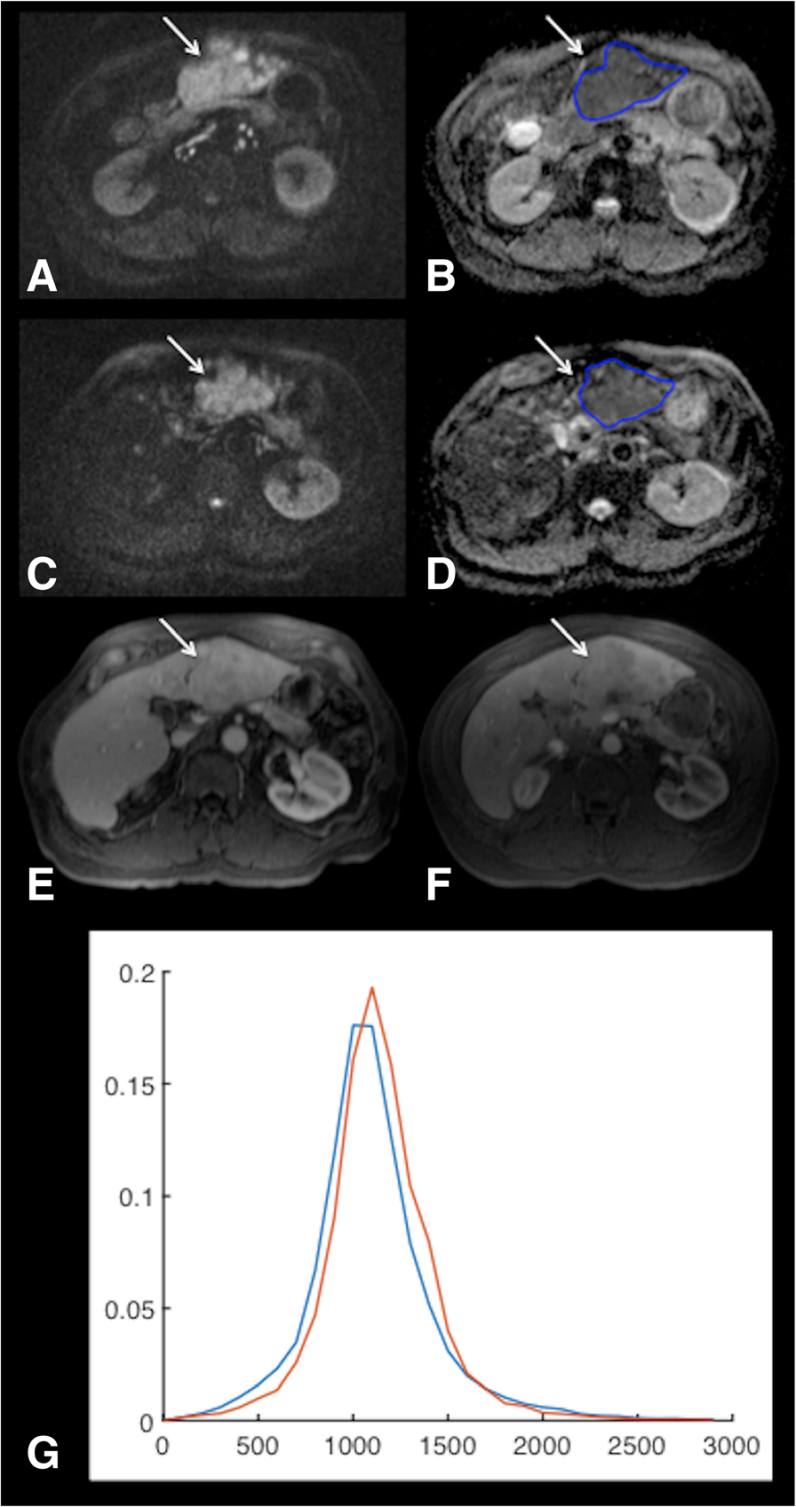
69-year-old male patient with HCV cirrhosis and HCC treated with radioembolization (RE). Pre-treatment MRI: HCC (arrows) demonstrates restricted diffusion on high b value DWI (b 800) (a) and ADC map (b). Post-treatment MRI at 6w post RE shows unchanged diffusion restriction on DWI (b 800) (c) with low ADC (d). Contrast-enhanced-T1 weighted images obtained during the portal-venous phase at baseline (e) and at 6 months (f) show stable disease at 6 months. Corresponding histogram distribution of vADC before (blue, vADC median 1.12 × 10^− 3^ mm^2^/s, vADC mean 1.12 × 10^− 3^ mm^2^/s) and 6w after RE (red, vADC median 1.17 × 10^− 3^ mm^2^/s, vADC mean 1.19 × 10^− 3^ mm^2^/s, ΔvADC median 4%, ΔvADC mean 3%) shows an almost identical distribution after RE (g)
